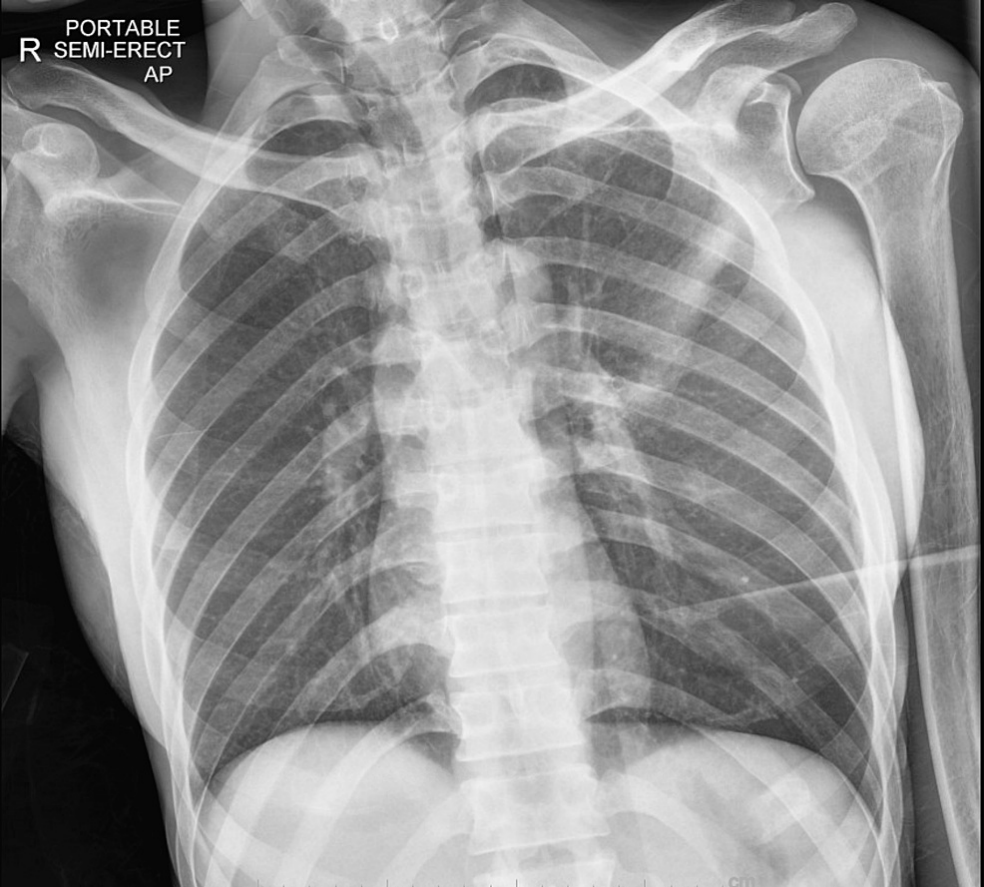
Chest X-ray at time of suspected IRIS, showing resolution of bilateral pulmonary infiltrate IRIS: immune reconstitution inflammatory syndrome
